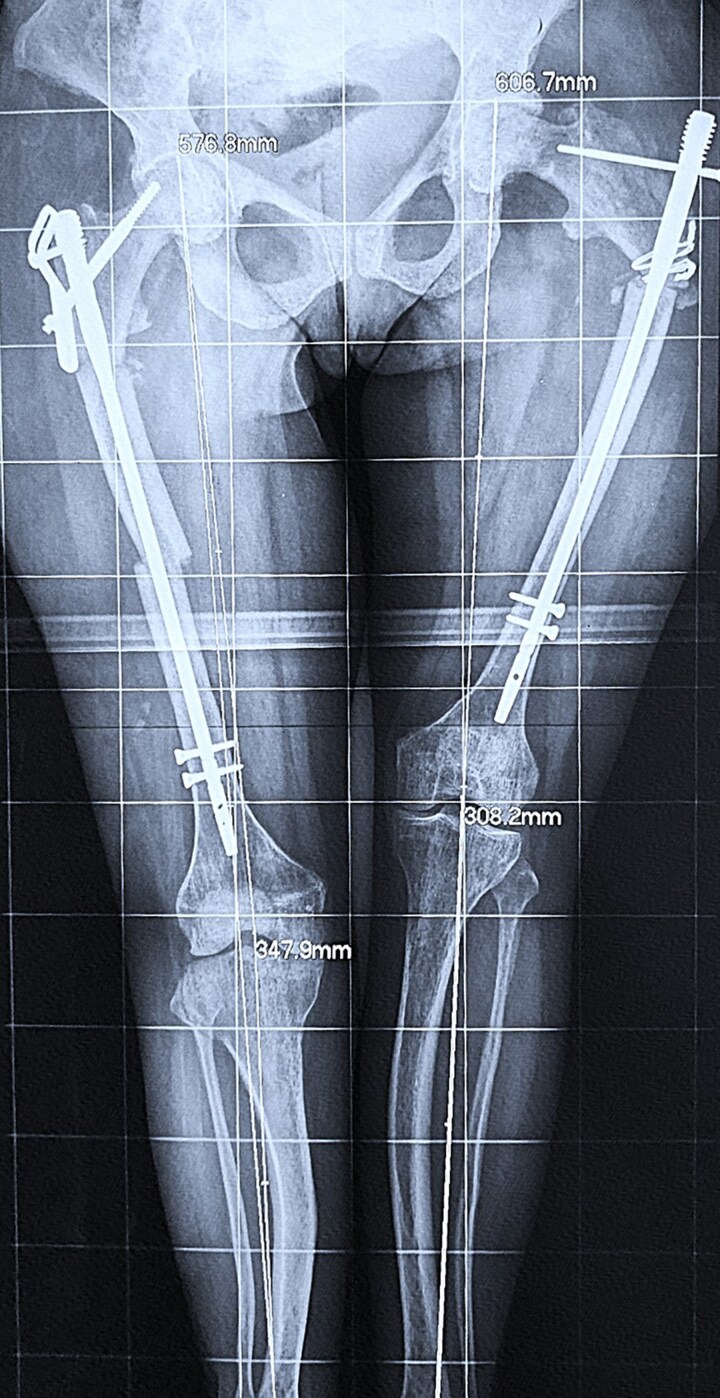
Postoperative radiographs after osteosynthesis.
